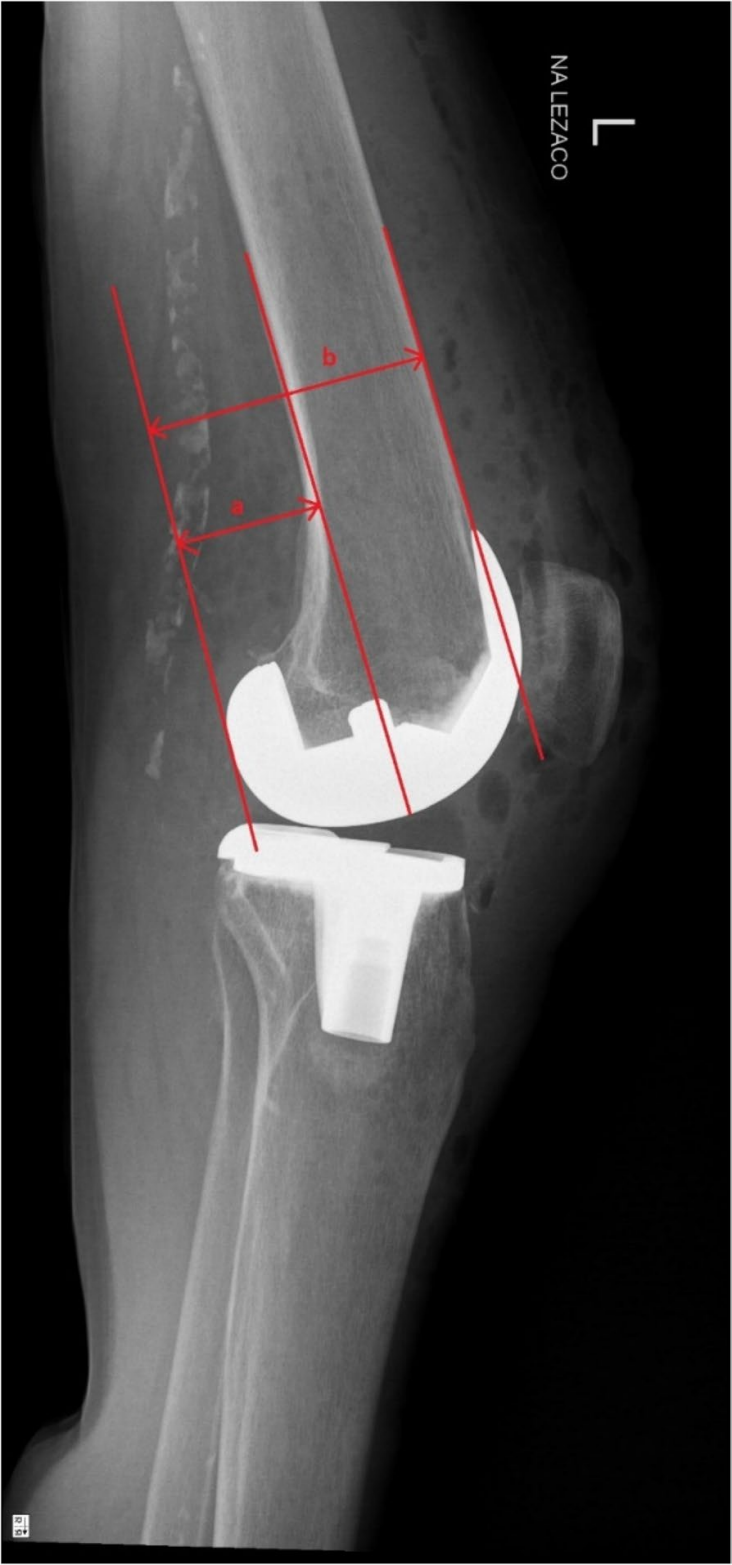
Measurement of postoperative posterior condylar offset ratio (a/b) on standard lateral x-ray
